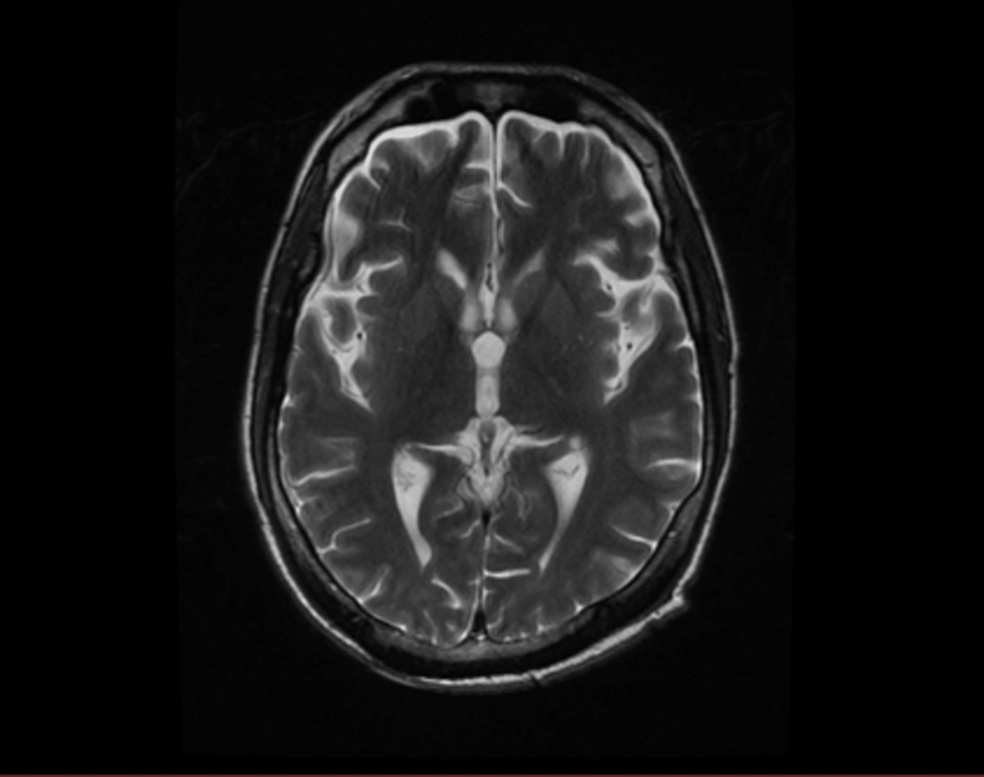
Brain MRI T2 showing significant resolution of the hyperintense lesions in the left internal capsule, both thalami and brainstem.
